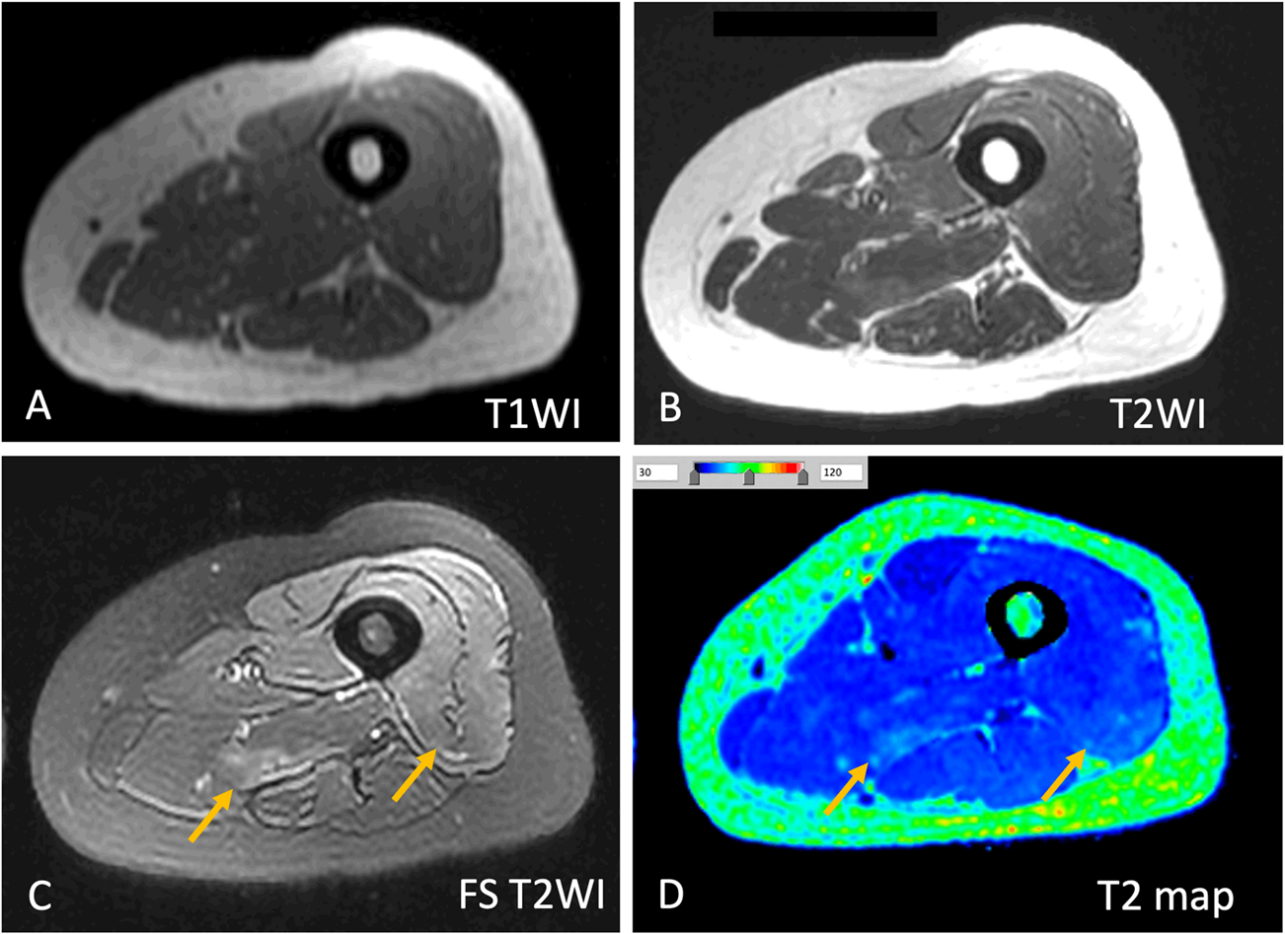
MRI of a 52-year-old female with dermatomyositis. (A) Axial T1-weighted image (T1WI), (B) T2WI, (C) fat-saturated (FS) T2WI, and (D) color-coded T2 map. The serum muscle enzymes of this patient were normal, while slight hyperintensity of quadriceps and adductor magnus (arrows) was noticed on FS T2WI which was further confirmed by an elevation of T2 value (44.9 ms) presenting as light blue in color-coded T2 map
